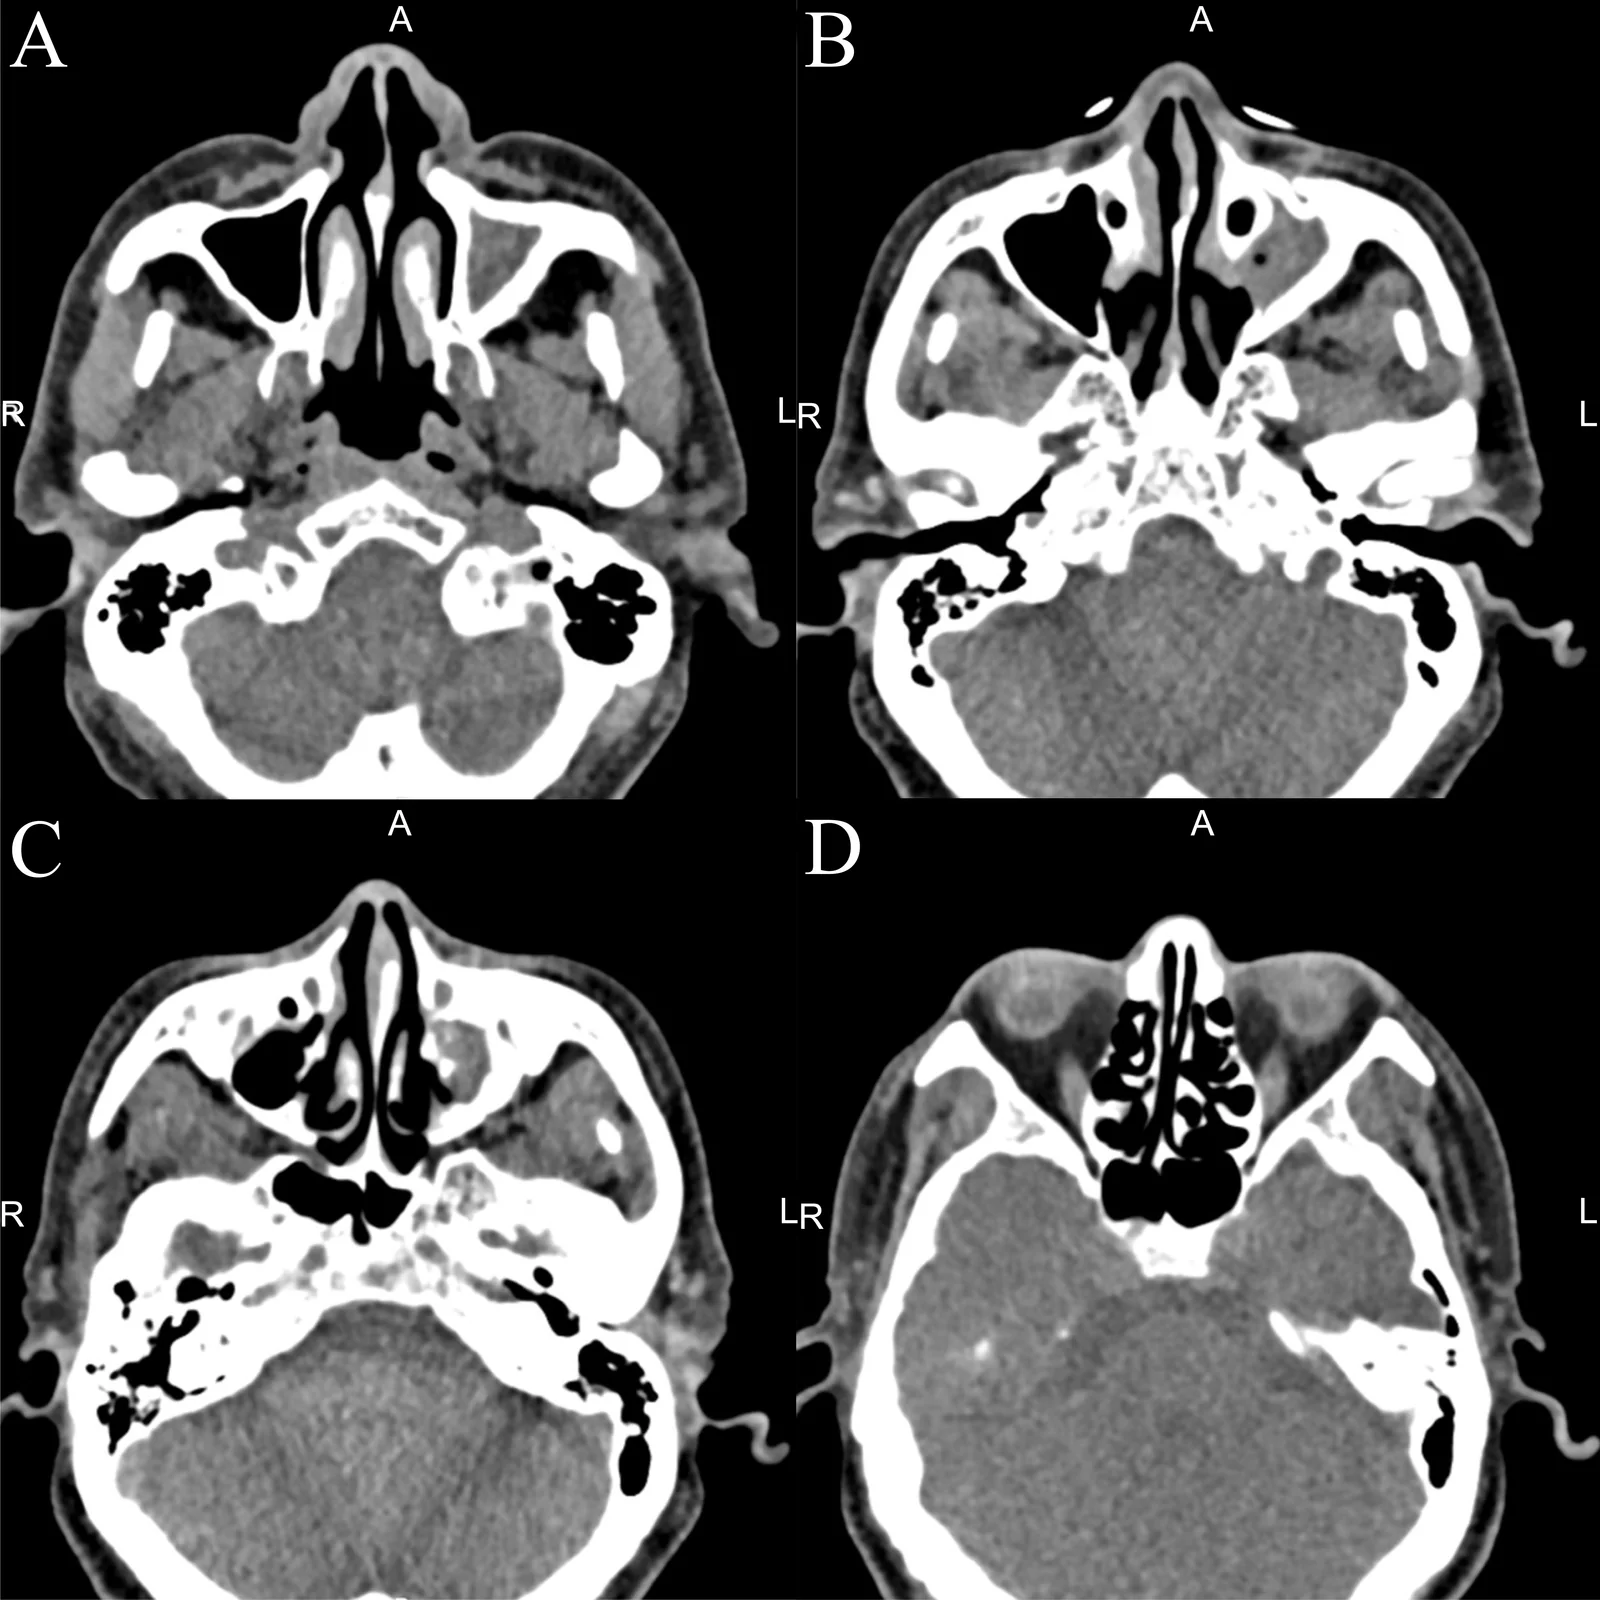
Sinus CT on 2023-09-01 showed high-density material within the left maxillary sinus with smooth bony walls and thickening of the sinus wall. The nasal septum was deviated to the left.
